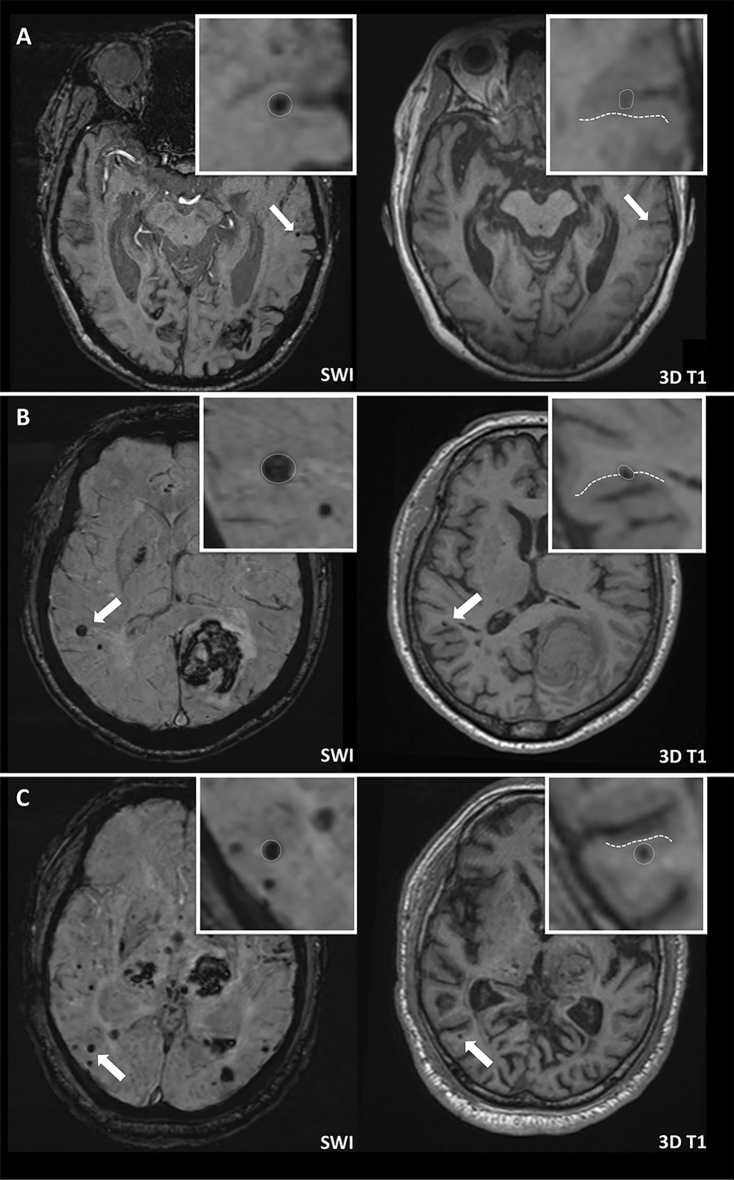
Categorization of lobar CMBs. The location of each lobar CMB was categorized using SWI and the corresponding 3D T1-weighted images. (A) Intracortical CMB: the lobar CMB is completely located in the gray matter of the cortex. (B) Juxtacortical CMB: the CMB is located on the border of a gray-white matter junction. (C) Subcortical CMB: the CMB is completely located in white matter. The borders of the cortex are outlined with dotted lines.
